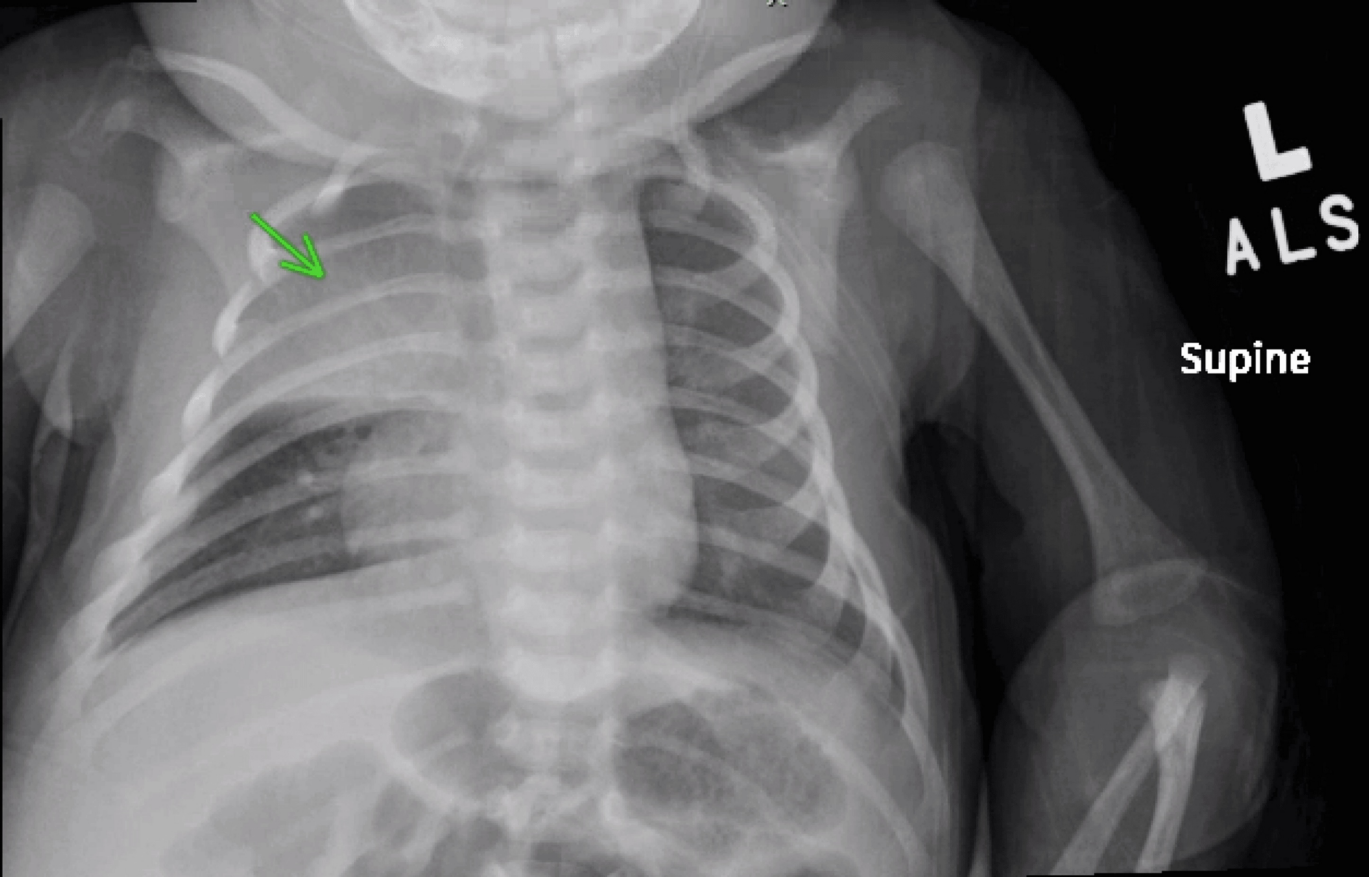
Chest X-ray on admission initially concerning for right upper lobe consolidation, later determined to represent a thymic shadow (arrow).
